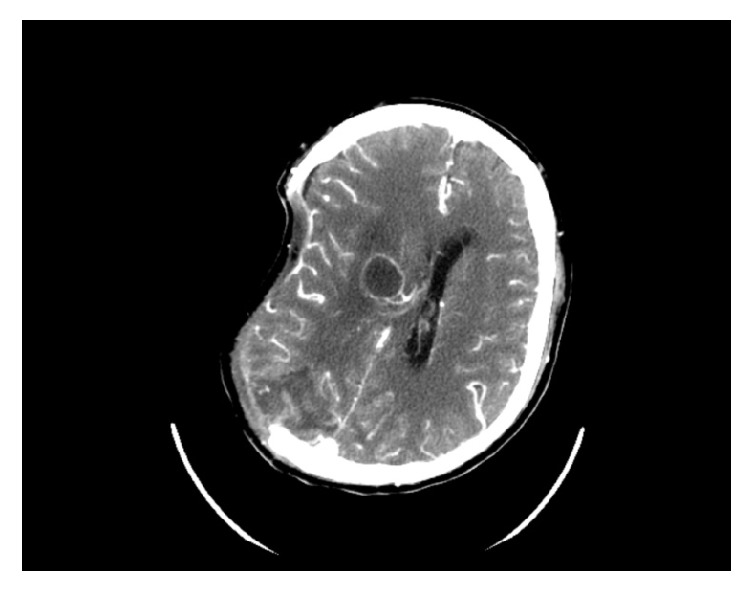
Contrast-enhanced CT of head 30 days after the initial ischemia showed a ring-enhancing cystic lesion in the right basal ganglia with perilesional oedema, which compressed right lateral ventricle with midline shift towards left suggestive of abscess.
